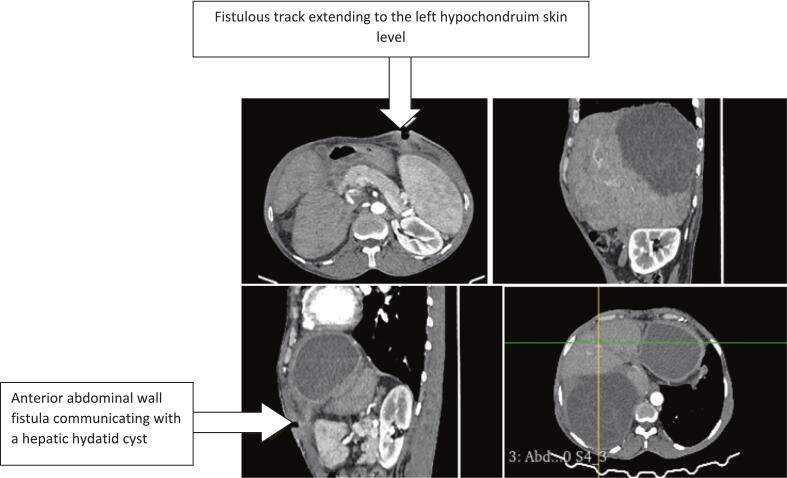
CT scan: subcutaneous rupture of a hydatid cyst of the left liver.
